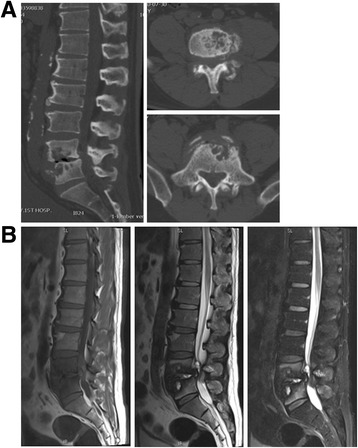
a. CT scan showed osteolytic lesion and new bone formation in the parts of the bodies of L4 and L5. b. Magnetic resonance imaging (MRI) revealed bone destruction and extraskeletal soft tissue formation posterior at the L4 vertebral body level corresponding with the stenotic area
